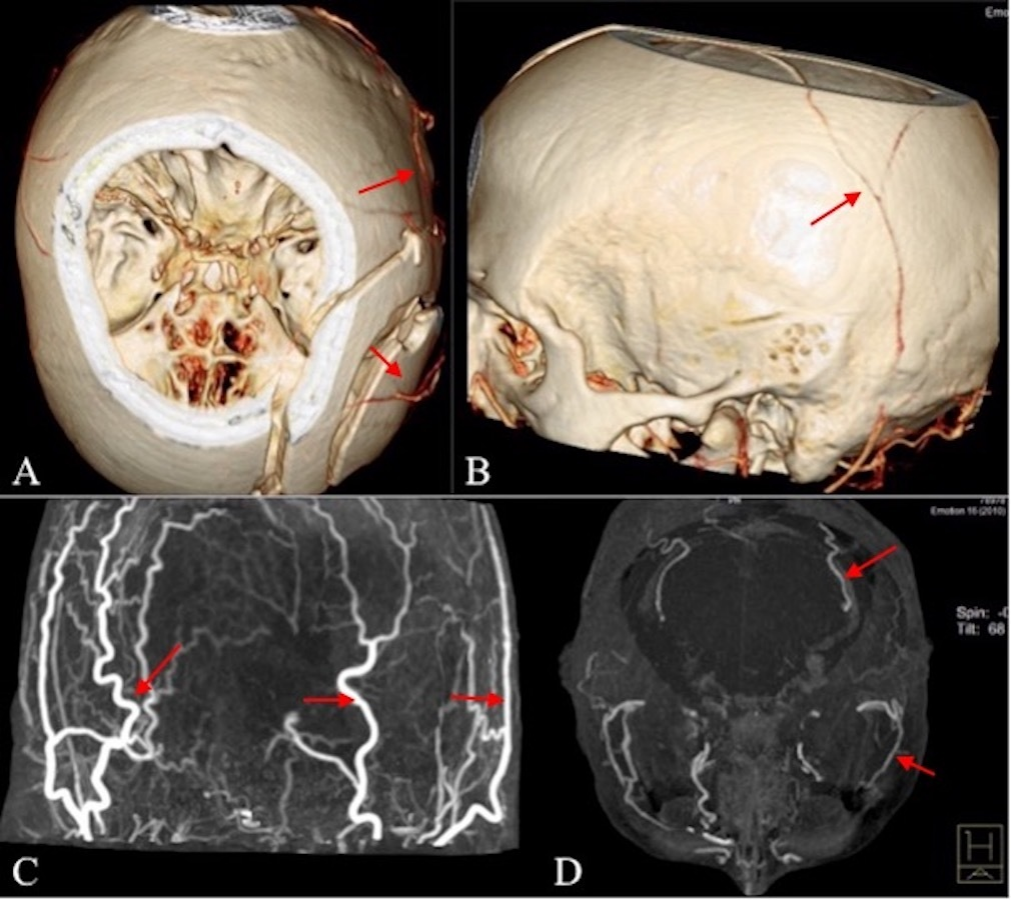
CT angiography with 3D reconstruction Images in four different patients show preservation of external cranial flow (A, B, C, D, red arrows) with no internal cranial opacification (A, C, D).
